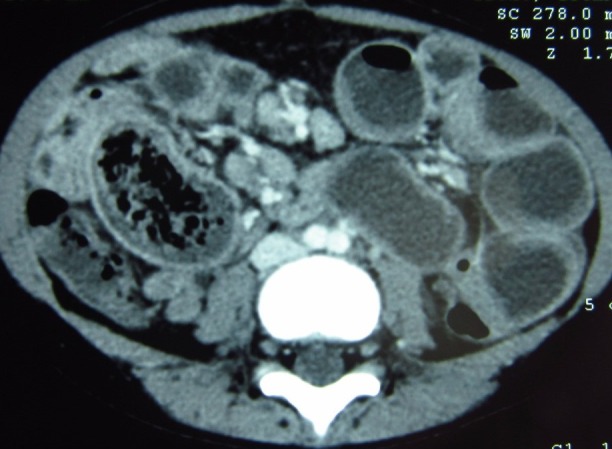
Figure 1: CT scan abdomen showing dilated fluid filled bowel loops with a heterogeneous mass in the small bowel lumen with spongiform appearance.
